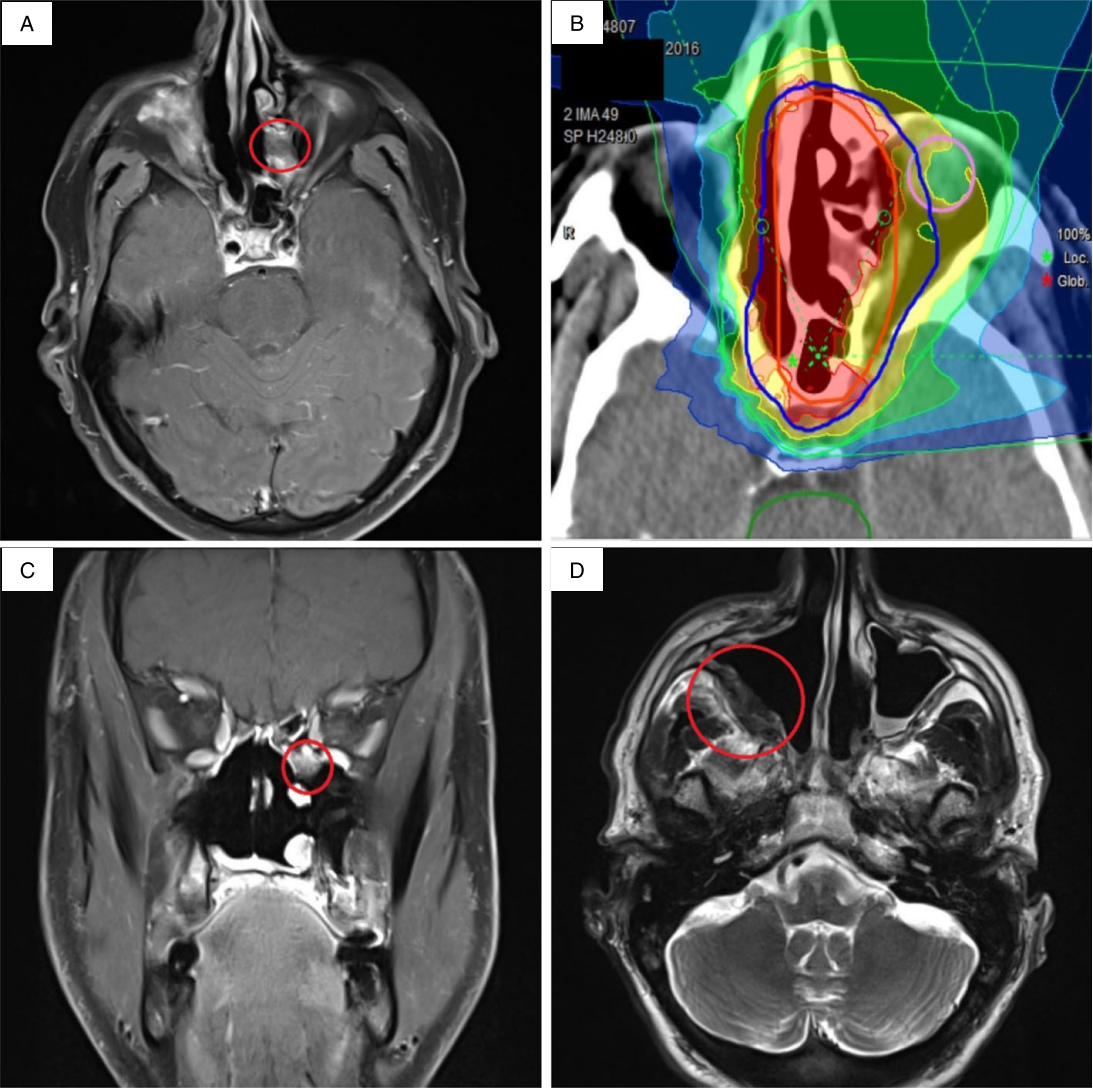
Shown are treatment and follow-up images of a 54 year old male patient with a recurrent small cell neuroendocrine carcinoma of the left paranasal sinus. After initial radiation treatment (66 Gy in 2 Gy fractions intensity-modulated radiation therapy) and three cycles of carboplatin and etoposide, the patient received re-irradiation with carbon ions (51 Gy (RBE) in 17 fractions) to the left paranasal sinus. The treatment planning T1 contrast-enhanced fat-suppressed magnetic resonance imaging (MRI) and the corresponding carbon ion treatment plan are shown in (a) and (b), respectively. The recurrent tumor was stable 24 months after treatment (c). Another 6 months later (30 months post-treatment), a recurrence developed in the contralateral paranasal sinus, shown as a T2w-hypointense tumor in the follow-up MRI (d)
